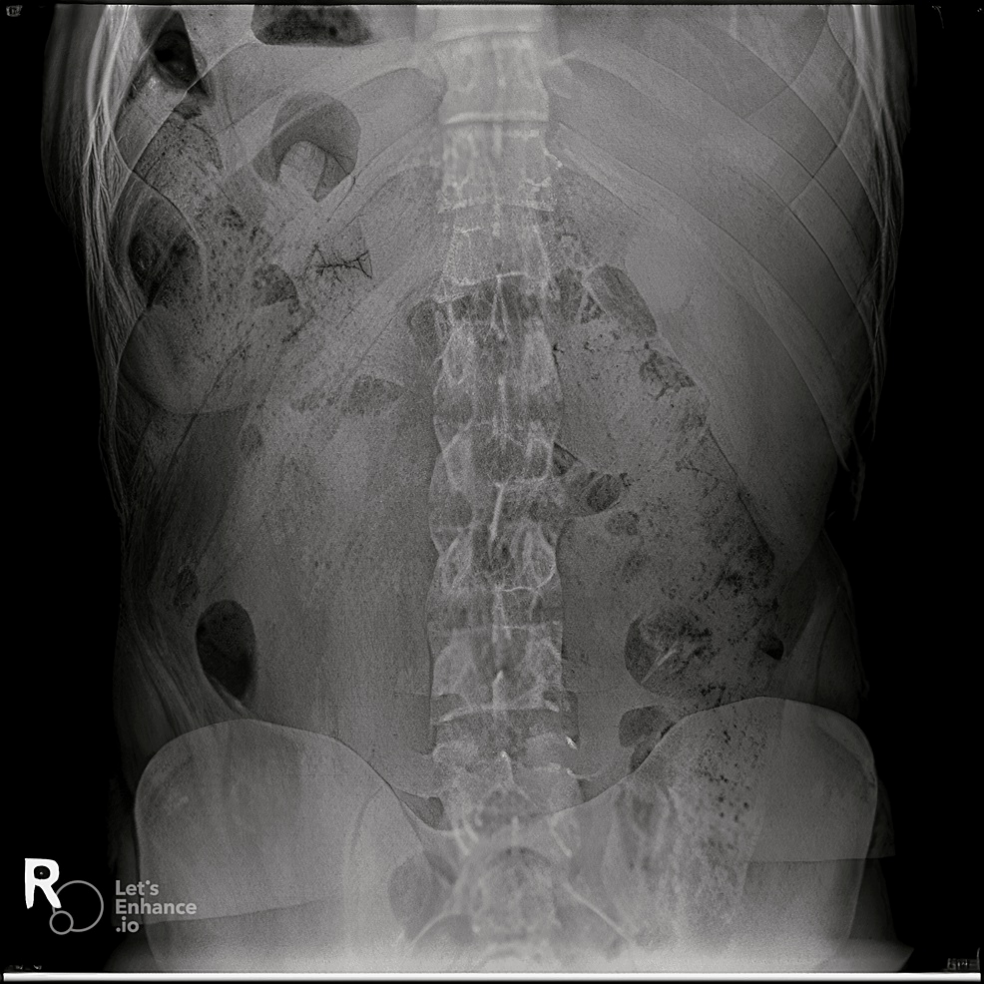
Abdominal X-ray showing mirror presentation of the intra-abdominal organs.
